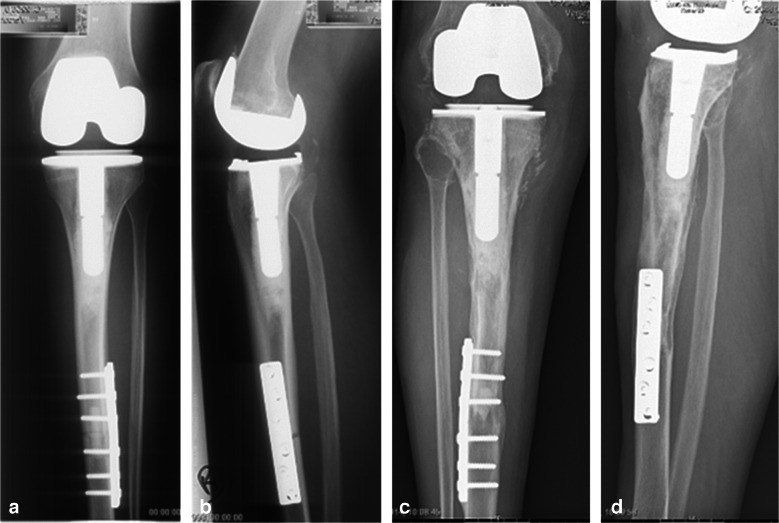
APC reconstruction of the proximal tibia following resection of a grade 1 chondrosarcoma. A and B: direct postoperative anteroposterior and lateral radiograph, showing reconstruction with a conventional knee replacement system, fixation of the allograft with a plate and additional intramedullary strut grafting. C and D: anteroposterior and lateral radiographs taken 18 years after the index procedure. The patient did not experience any complications. There is sound incorporation of the allograft and there are no signs of implant loosening
